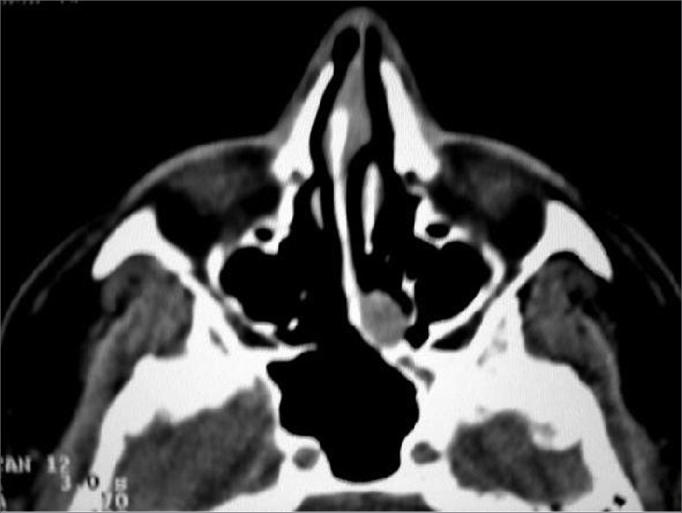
Preoperative CT scan: mucosal thickening in the sphenoethmoidal recess matching the endoscopic finding.
